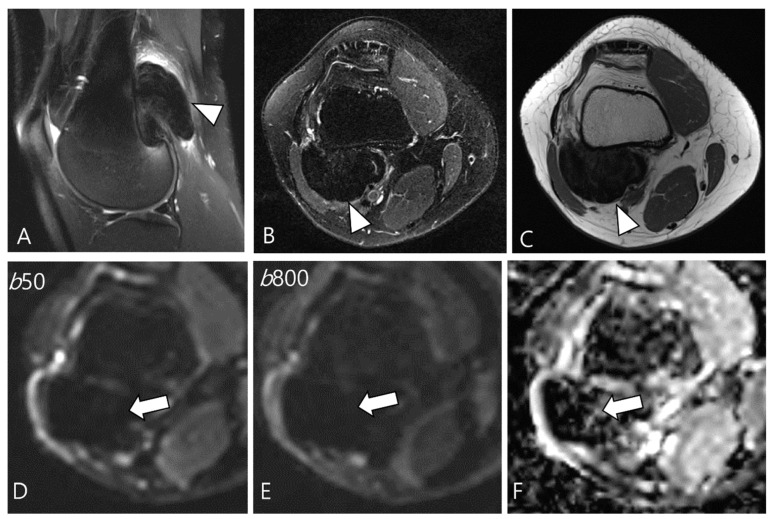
A 29-year-old female with D-TSGCT in the distal femur. (A,B) Sagittal and axial fat-suppressed T2WIs show a low SI mass at the popliteal fossa (arrowheads). (C) Axial T1WI also shows low SI due to extensive hemosiderin deposition (arrowhead). (D,E) The mass shows low SI on low and high b-value images of DWI due to hemosiderin deposition (arrows). (F) The mass creates a pseudo-low ADC value on the ADC map (arrow), suggesting T2 black-out effect.
